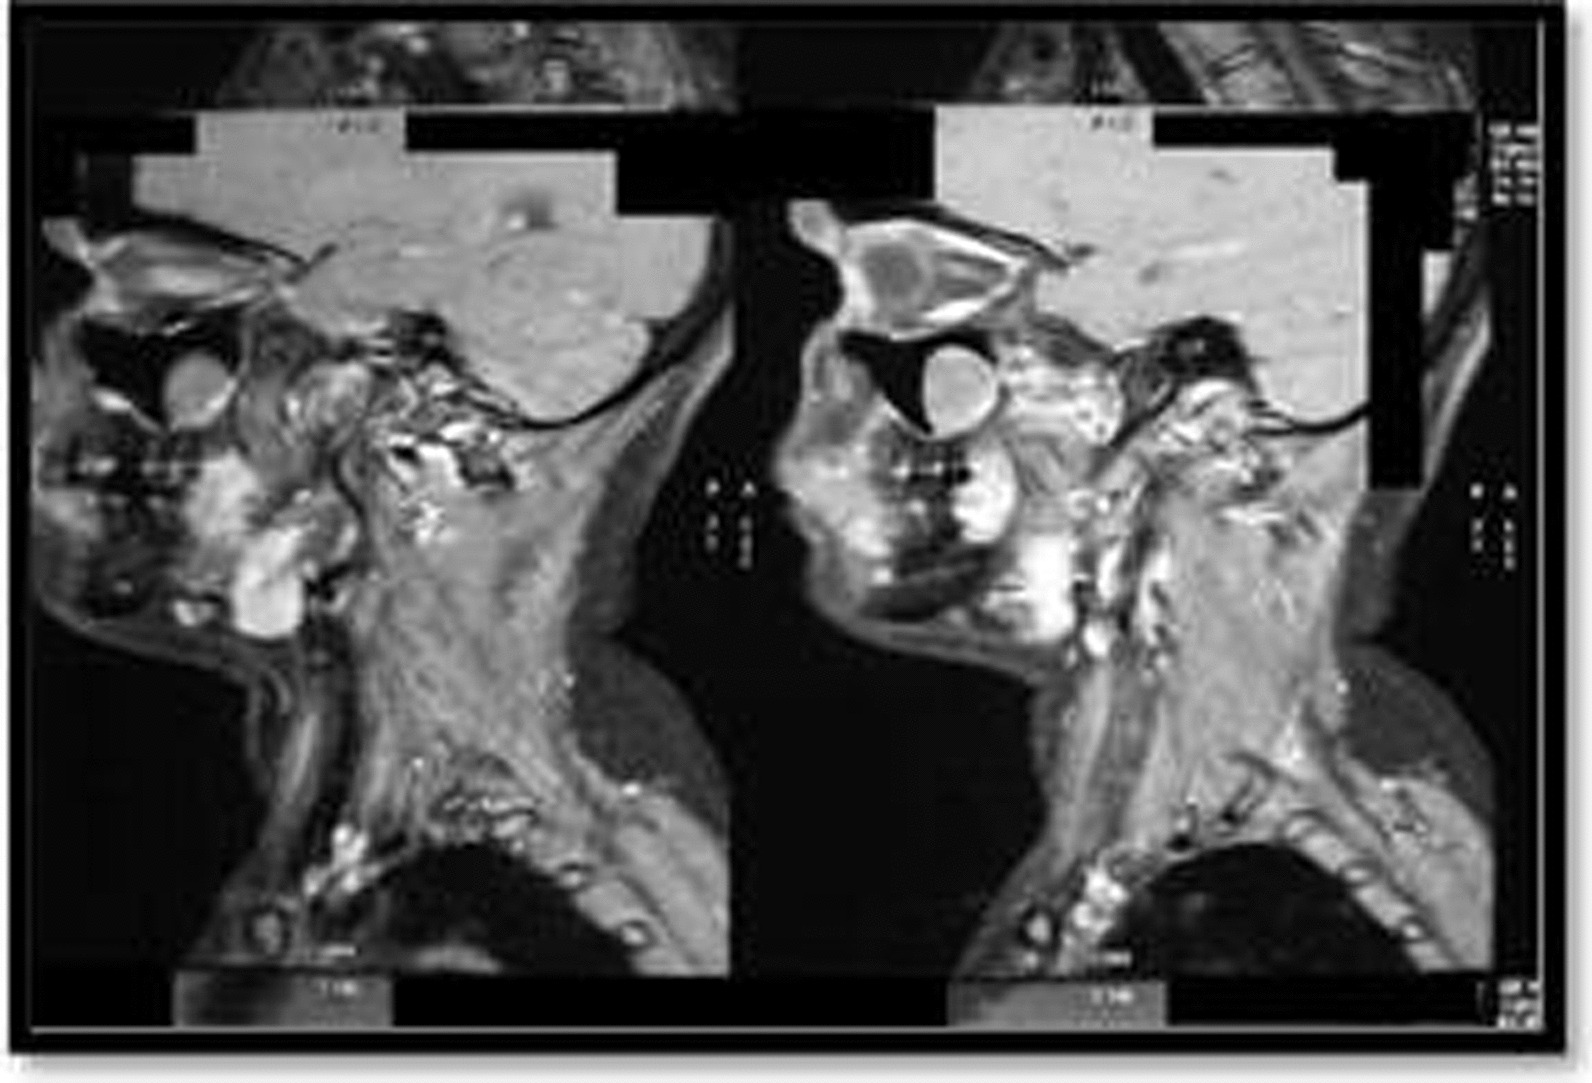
Magnetic resonance imaging (MRI) scan showing lesion over the right premaxillary region
